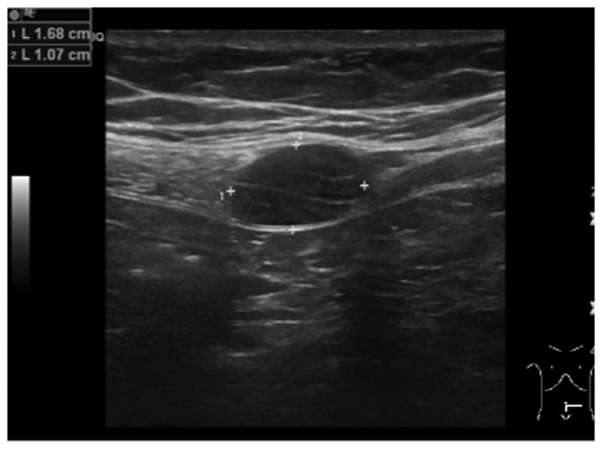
Ultrasound examination revealing a regular oval-shaped hypoechoic mass of the left abdominal wall, with internal linear hyperechoic structures, that is well-demarcated from the sourrounding structures.
